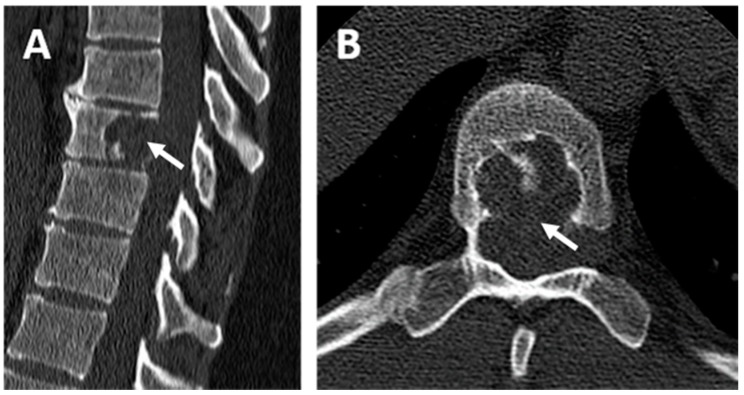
Computed tomography scan (CT scan) of the patient’s spine. (A) Dorsal CT scan in the sagittal plane and (B) dorsal CT scan in the axial plane centered on the ninth dorsal vertebra (T9). Osteolytic lesion containing septa centered on the body of the ninth dorsal vertebra (white arrow) with lysis of the posterior wall without osteo-condensation and fluid density.
